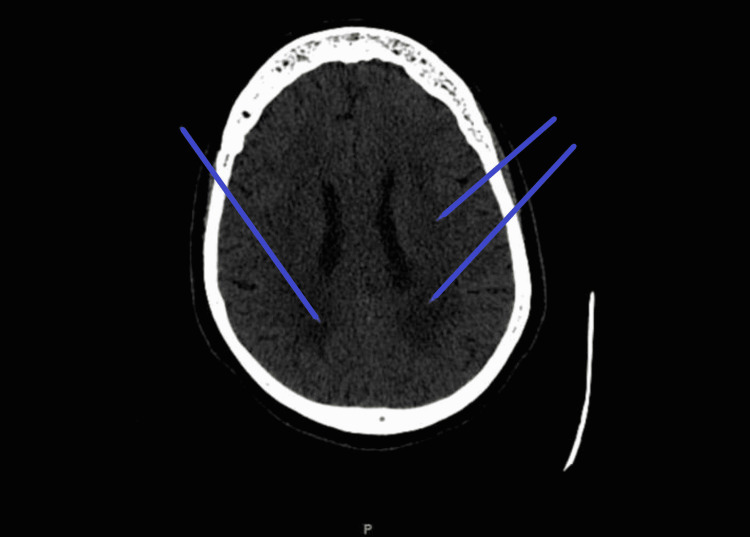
CT brain non-contrast: Transversal view showing greater than typical for age ischemic white matter disease.
